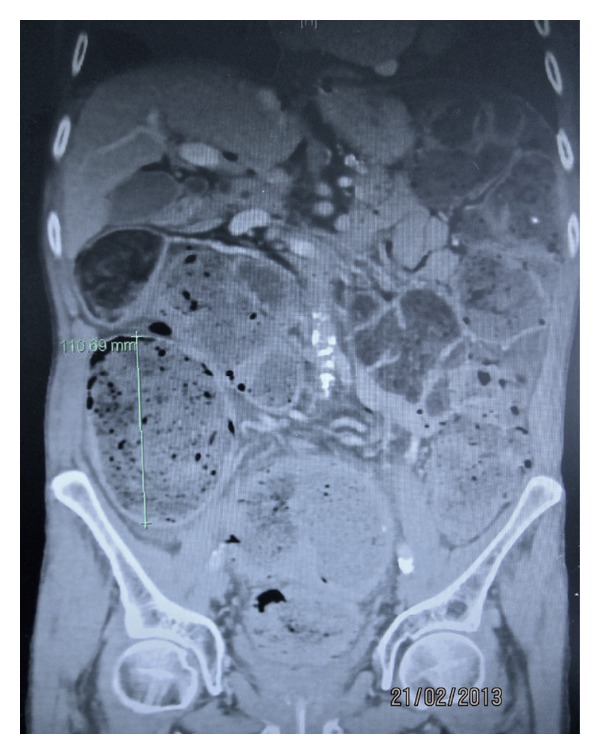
CT abdomen and pelvis demonstrating gross faecal loading and colonic dilatation.
